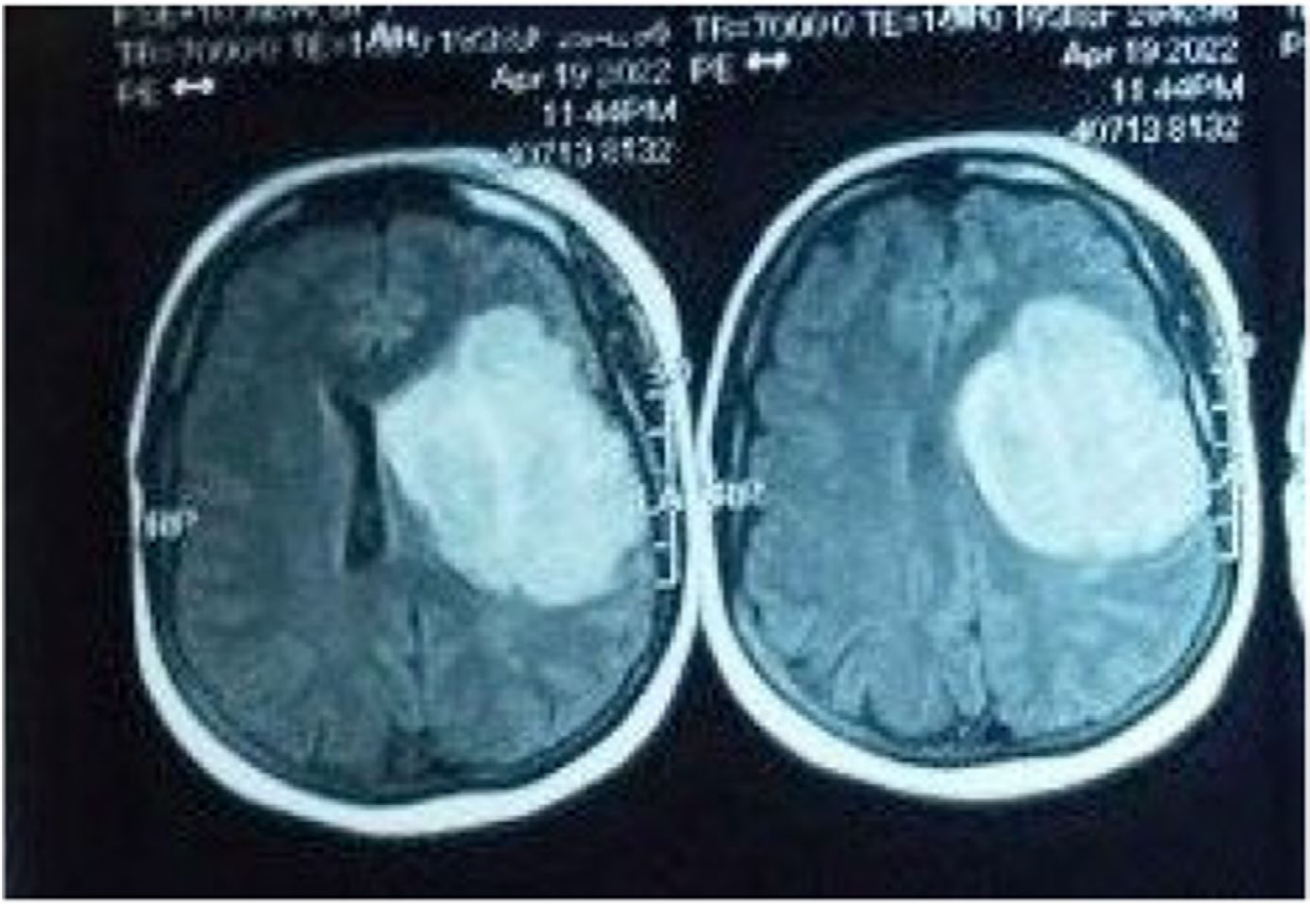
FLAIR showing high signal intensity.
